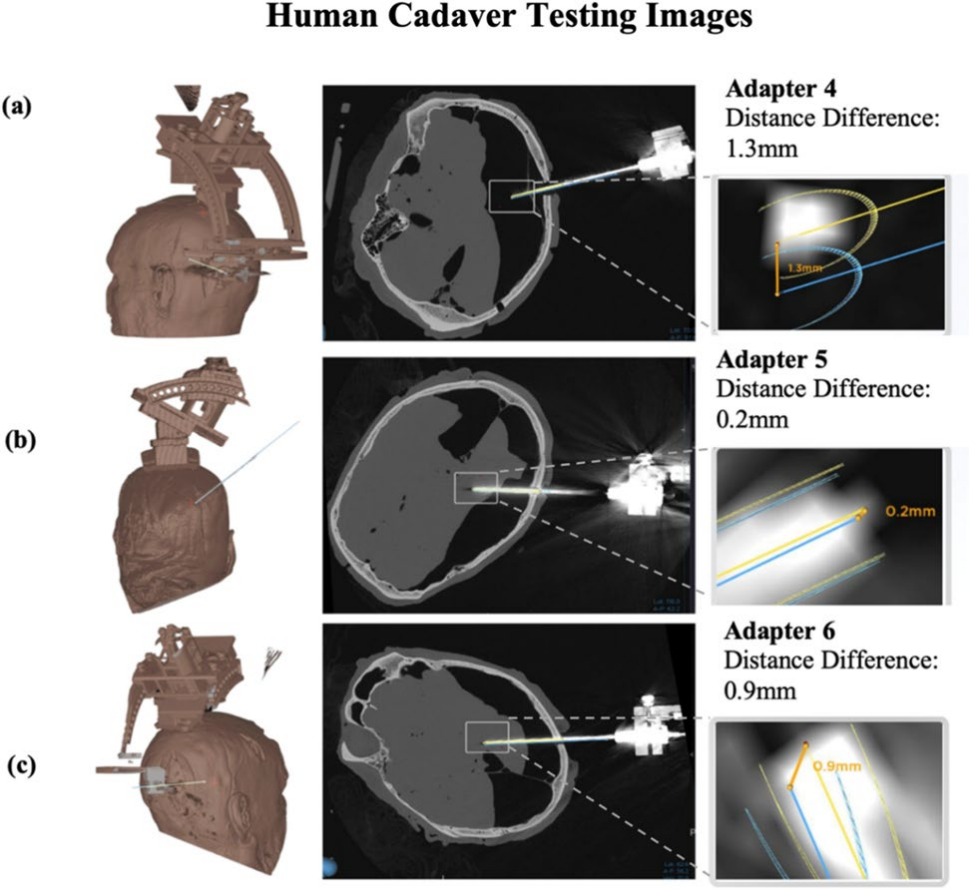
CT Images extrapolated from Medtronic™ Stealth Station. a CT image of adapter 4 shown in a 3-dimensional and axial view. The blue plan is the expected implantation location and trajectory of the targeting probe. The yellow line is the actual implantation location and trajectory. The magnified portion indicates the distance measured between the yellow and blue line indicating its accuracy. b CT image of adapter 5 shown also in a 3-dimensional and trajectory view. Same as adapter 4, the magnified portion shows the difference in distance indicating the accuracy. c CT image of adapter 6 is shown as a 3-dimensional and trajectory view. The accuracy can also be shown in the magnified portion
